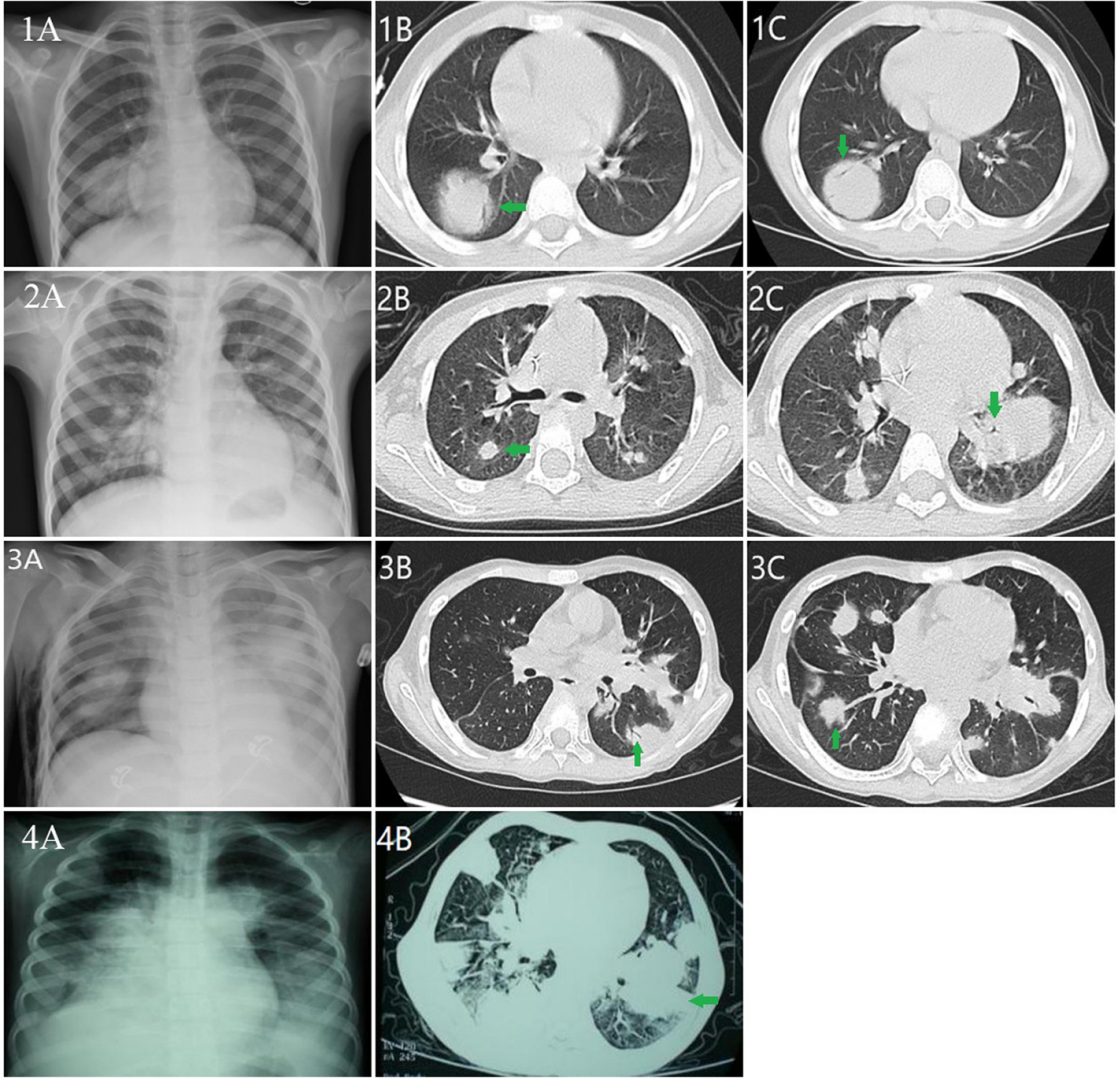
Chest film and CT scan shows a circular consolidation (3.7 × 3.1 cm) with poorly defined borders in the right lower lobe. An air bronchogram is readily seen (1a-c, Patient 1); Chest film and CT image shows multiple nodular masslike areas of consolidation with halo signs and air bronchograms in both lungs (2a-c, Patient 2 and 3a-c, Patient 3); Chest film and CT image shows multiple nodular masslike areas of consolidation with halo signs in both lungs (4a-b, Patient 4)
